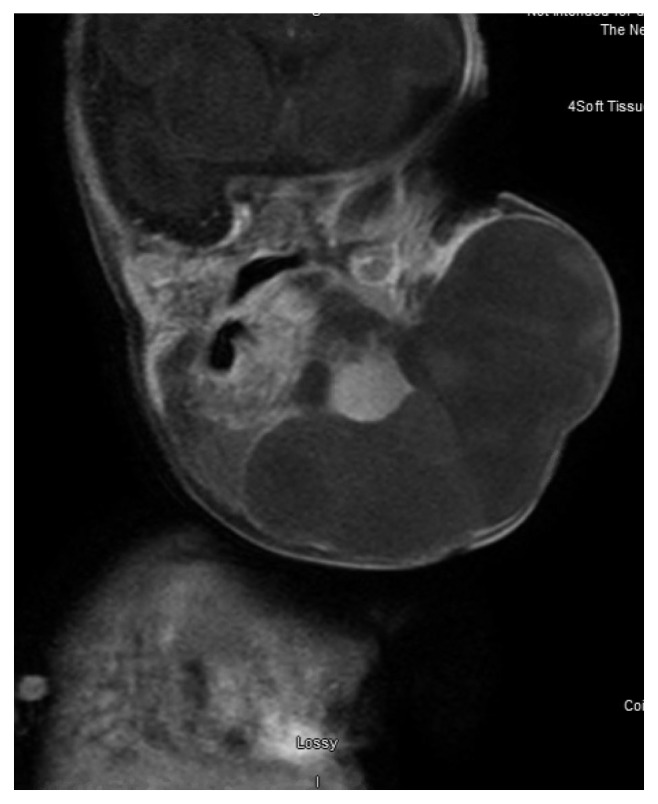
T1+ contrast coronal MRI demonstrates a large mixed-cystic LM extending from the left neck into the chest with recent hemorrhage, measuring 8.1 × 5.7 × 5.5 cm.
